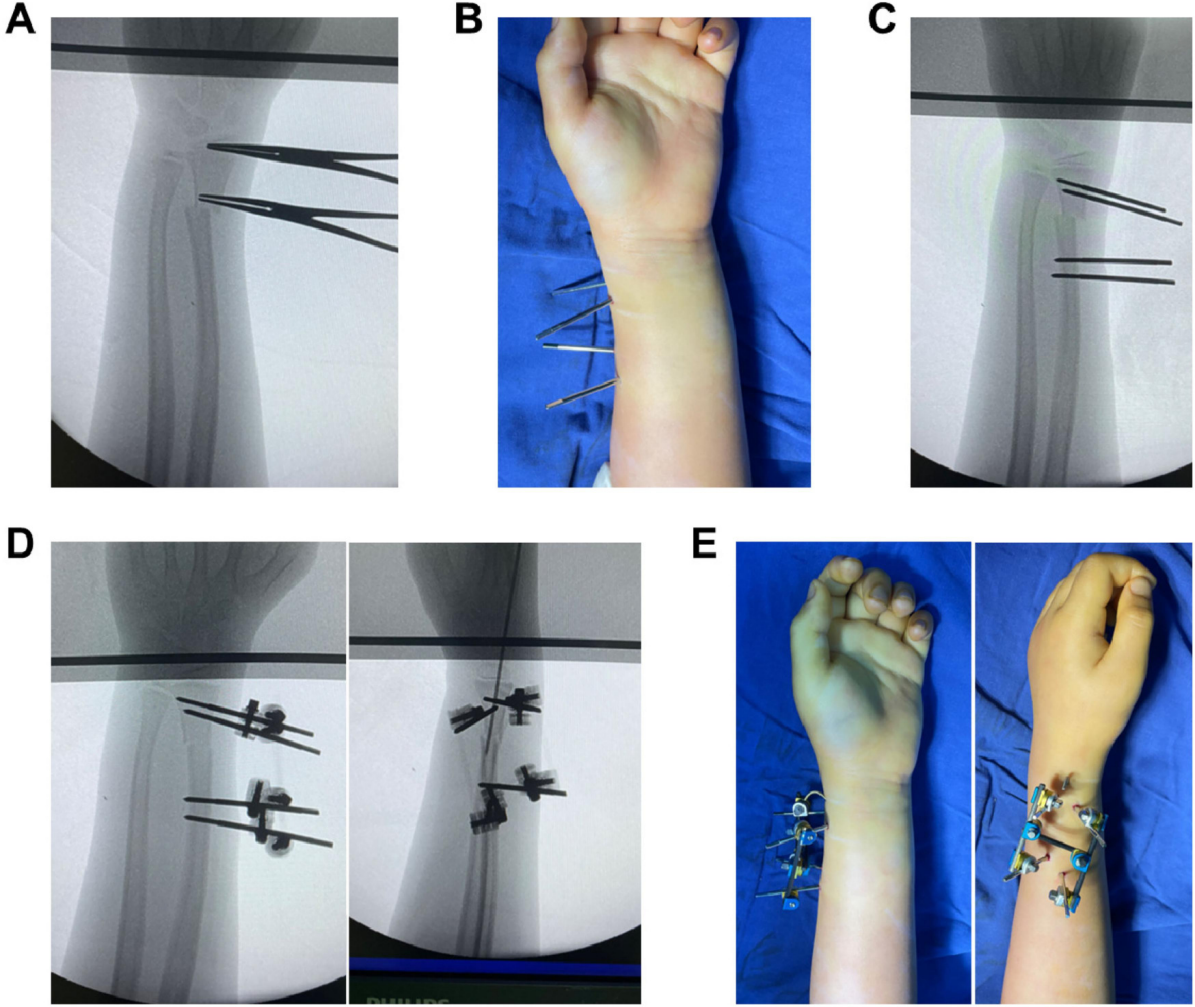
External fixation procedure for pediatric distal radius fractures. (A) x-ray localization of fracture and epiphysis. (B) Bilateral 2.5 mm pin insertion through stab incision. (C) Fixation-assisted closed reduction. (D) Supplemental K-wire for stability. (E) Minimal scarring for surface.
